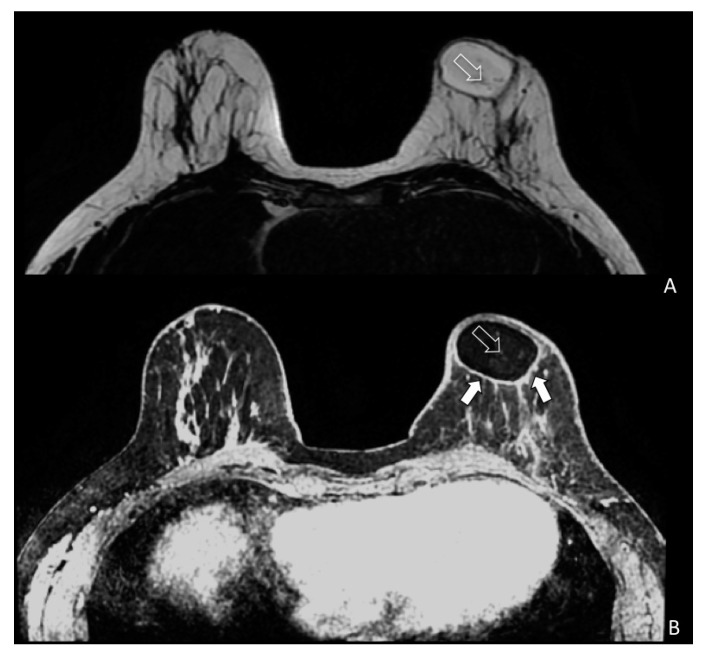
Magnetic resonance imaging (MRI) of a patient (SG) treated with EF-e-A. (A) Axial MRI T2-weighted turbo spin echo image of both breasts. Fat is imaged with hyper-intense signal and glandular tissue with the matrix is characterized by hypo-intense signal. In the left breast is showed an oval area of hyper-intense signal representing the fat grafting. Very small vessels are depicted in the area (white empty arrow); (B) Bilateral three-dimensional T1-weighted spoiled turbo gradient echo image after contrast media administration VIBRANT sequence showed contrast uptake of the glandular tissue and of the fat graft boundary (white arrows). In this sequence, fat graft is characterized by hypo-intense signal due to the fat saturation pulse. In the fat graft, small vessels characterized by contrast media uptake are confirmed (white empty arrow).
